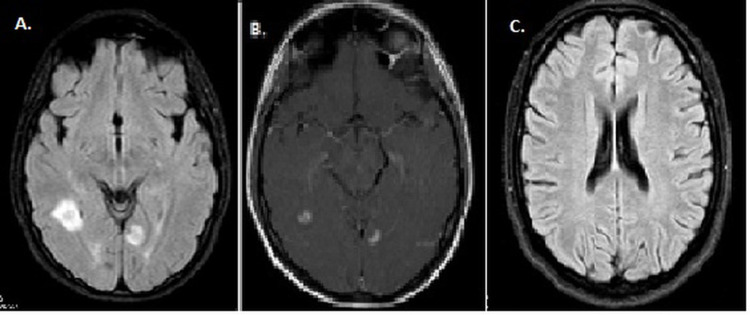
Case 1 Brain MRI revealed hyperintense periventricular and juxtacortical lesions in an axial FLAIR sequence (A) and contrast enhancements in axial T1 sequence (B). After two months, it was observed that the lesions regressed in the FLAIR sequence (C).
